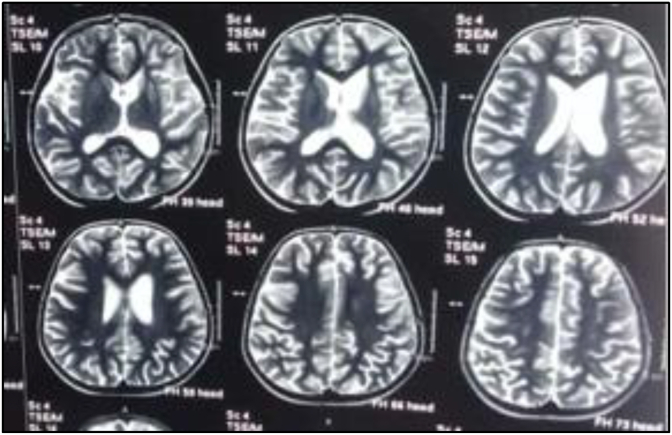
MRI T2 axial reveals atrophy in left Caudate and putamen nuclei.
